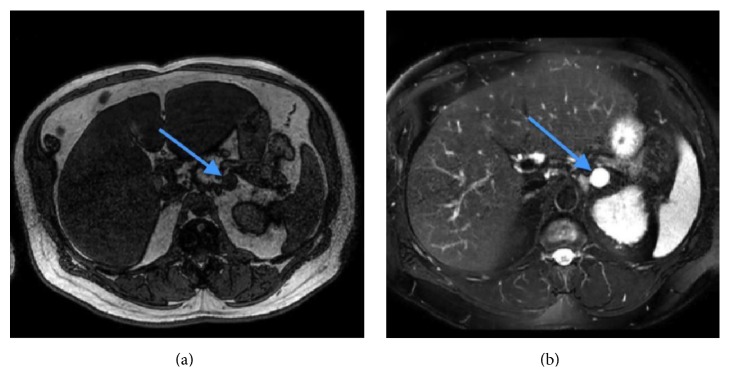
MRI of the adrenals. Within the lateral limb of the left adrenal gland, there is a 2.2 × 2.2 cm lesion (blue arrows) with uniformly high signal intensity on T2-weighted sequences (b) and low signal on T1-weighted images (a) with no significant loss of signal on opposed phase relative to in phase images.
